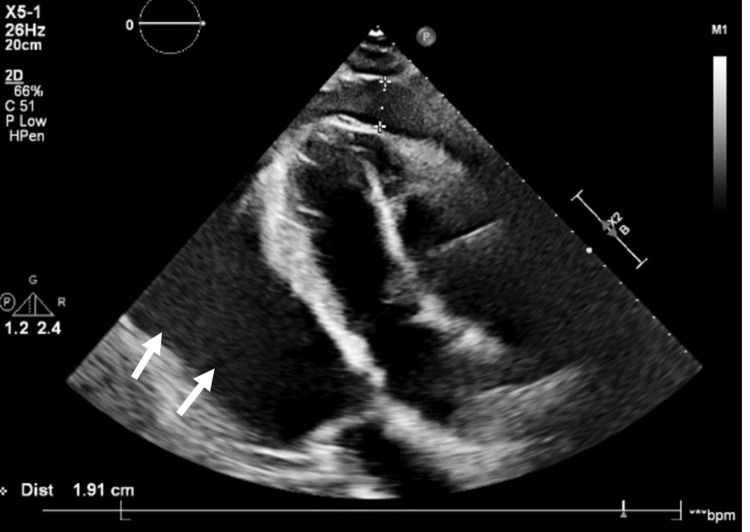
Large circumferential pericardial effusion surrounding the heart, as seen in the parasternal long axis.
